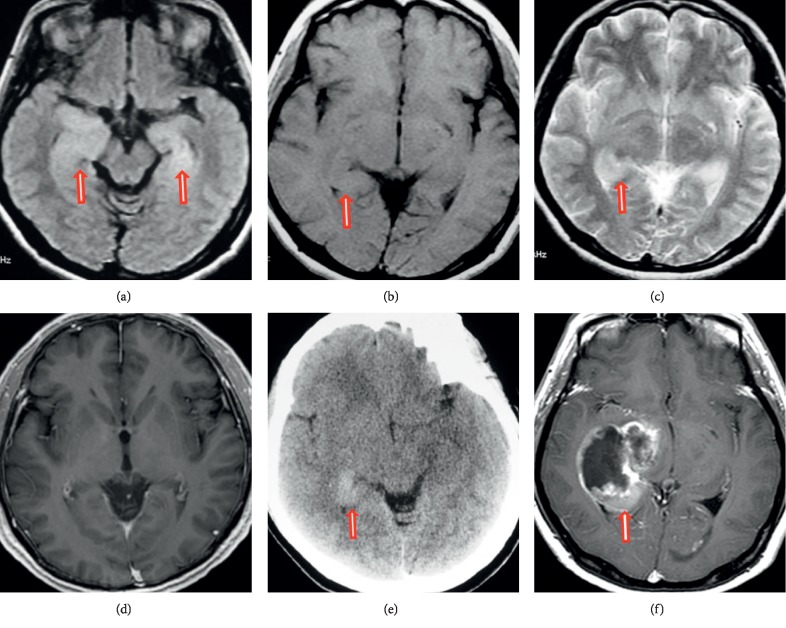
Magnetic resonance and computed tomography images of the brain. (a) Axial fluid-attenuated inversion recovery (FLAIR), (b) T1-weighted, (c) T2-weighted, and (d) contrast-enhanced magnetic resonance images show a poorly demarcated lesion involving the bilateral temporal lobe and hippocampus. The lesion shows homogeneous isointensity on T1-weighted imaging and homogeneous hyperintensity on T2-weighted and FLAIR imaging without perilesional edema or mass edema. The diffusion-weighted image shows an isointense lesion with a hyperintense region. Postcontrast magnetic resonance imaging shows no enhancement. (e) Axial-view head-window unenhanced computed tomography images of the head show a hyperattenuated region (arrow). (f) At the 7-week follow-up, the contrast-enhanced T1-weighted image shows a markedly larger ring and heterogeneously enhanced mass.
